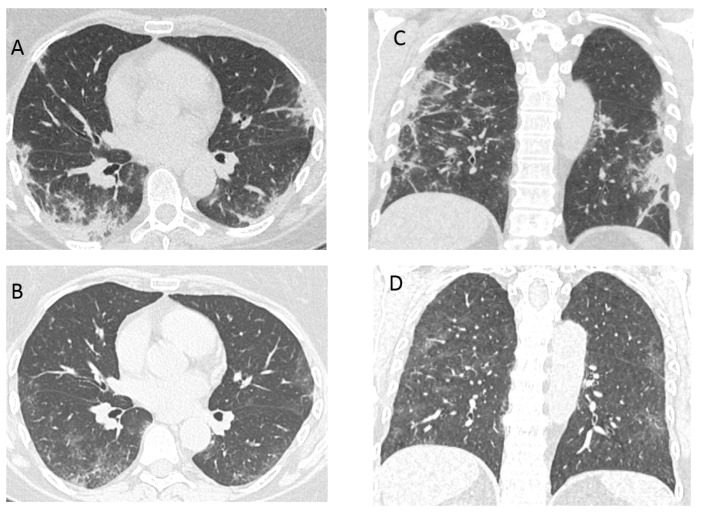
(A,C) Computed tomography (CT) of a 58-year-old woman, 21 days after COVID-19 pneumonia diagnosis, treated at home, with persistent and deteriorating respiratory symptoms. Peripheral consolidation with air bronchograms, reticular changes and small areas of ground glass opacities (organizing pneumonia). (B,D) CT after 4 weeks of treatment with systemic glucocorticoids—resorption of consolidation with the fibrotic component (reticular changes, bronchiectasis).
